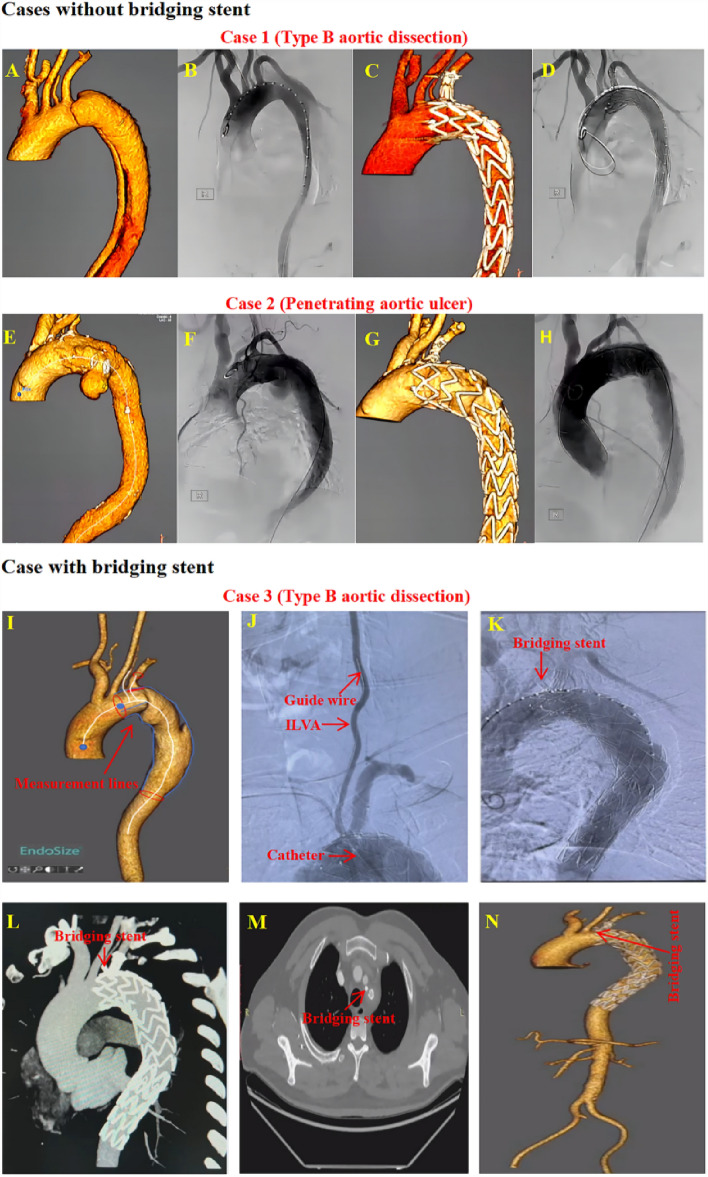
Presentations of outcomes using PMF on Castor branched stent technique to reconstruct ILVAs. Two cases without bridging stent implantation: (A,E) CTA display the aortic pathologies. (B,F) DSA show the aortic pathologies. (C,G) CTA display the perfect procedure utcomes. (D,H) DSA show the perfect procedure outcomes. One case with bridging stent implantation: (I) Measurement lines of preoperative CTA using EndoSize software (version 3.1.36 (5bb19e4)). (J) Intraoperative implementation of rebuilding ILVA. (K) DSA display the perfect procedure outcomes. (L–N) CTA display the perfect procedure outcomes. *PMF* physician modified fenestration, *ILVA* isolated left vertebral artery, *CTA* computed tomography angiography, *DSA* digital subtraction angiography.
